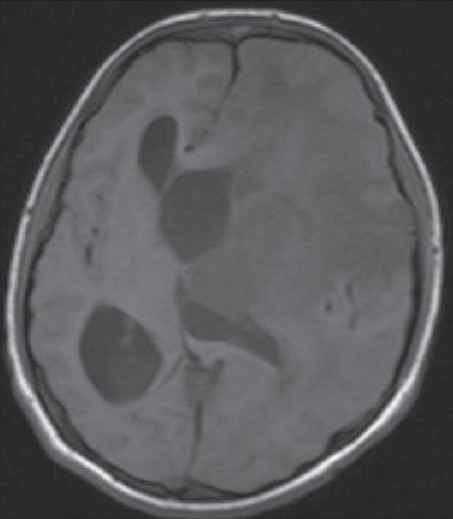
Axial section of the T1-weighted magnetic resonance imaging image of the brain showing the tumor with a midline shift
